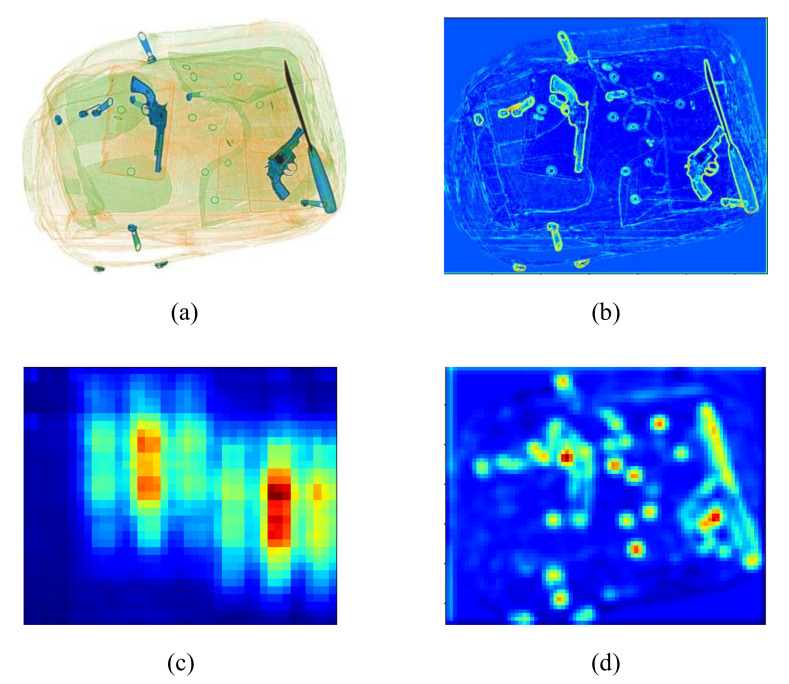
Original X-ray image and feature outputs from different convolutional layers from the backbone of RFB Net. (a) is the original image, (b) is the 2nd layer output, (c) is the 15th layer output and (d) is the 9th layer output.
